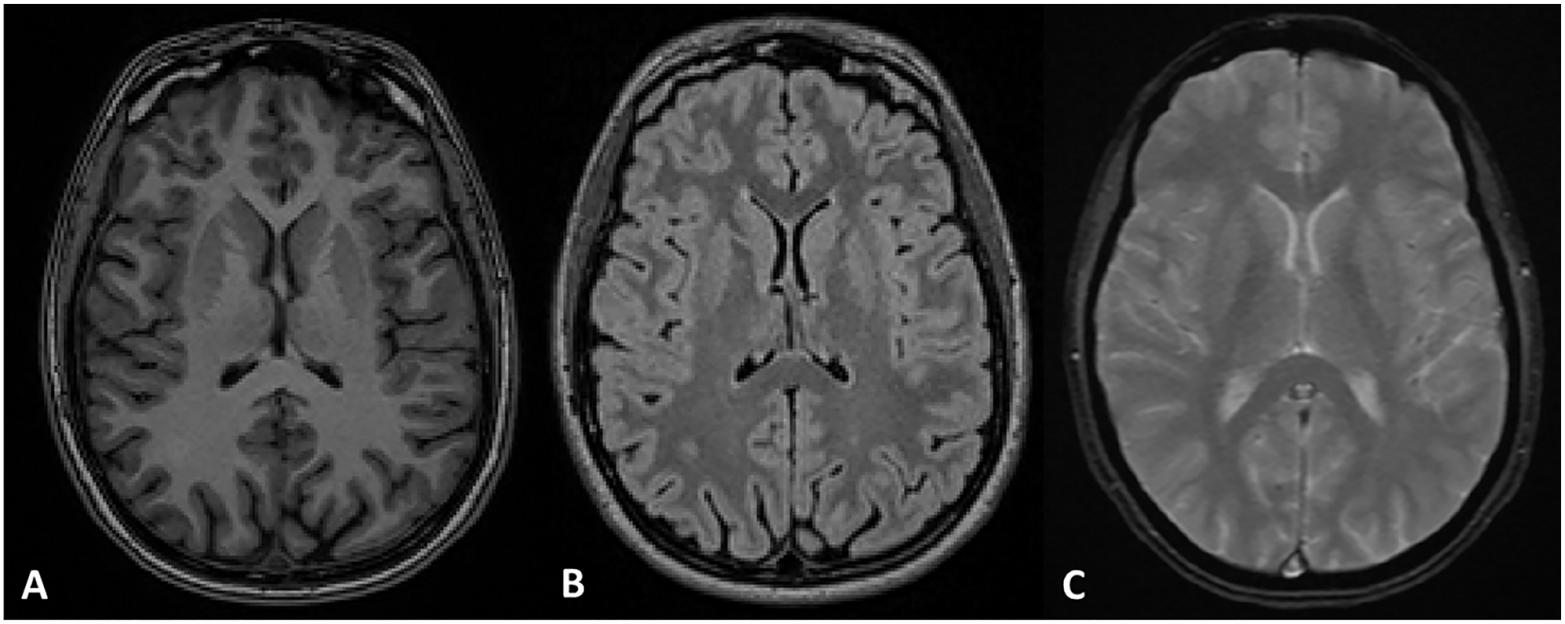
Examples of images obtained from the MRI protocol. A) Three-dimensional T1-weighted image; B) Three-dimensional dark-fluid T2-weighted image; C) two-dimensional axial T2*-weighted image.
